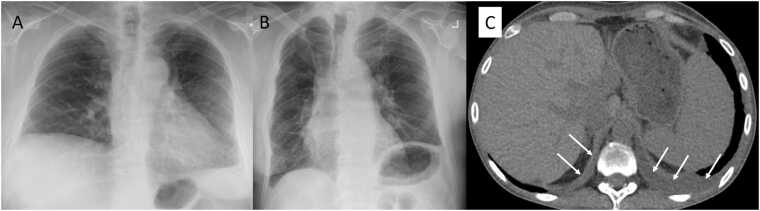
Representative images of diaphragmatic elevation and pleural effusion in patients with restrictive ventilatory defects without RLO. (A) CXR image demonstrating right hemidiaphragm elevation of more than 4 cm higher than the left hemidiaphragm, with a patient still alive at 33 months after CLAD diagnosis. (B) CXR image demonstrating left hemidiaphragm elevation higher than the right hemidiaphragm, with a patient still alive at 32 months after CLAD diagnosis. (C) CT image showing predominantly left-sided pleural effusion (white arrows). The patient died 28 months after CLAD diagnosis. CLAD, chronic lung allograft dysfunction; CT, computed tomography; CXR, chest x-ray; RAS, restrictive allograft syndrome; RLO, RAS-like opacities.
